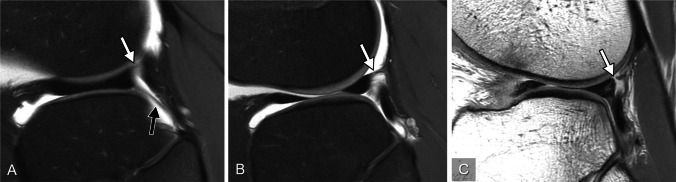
Popliteomeniscal fascicles. Sagittal T2-weighted fat-suppressed MR arthrographic images in a 15-year-old girl (A, B) show normal inferior (black arrow) and superior (white arrow) popliteomeniscal fascicles. Sagittal proton-density-weighted MRI of a 20-year-old man (C) with an acute ACL tear shows a torn superior popliteomeniscal fascicle (arrow); note associated anterior tibial translation owing to ACL deficiency
